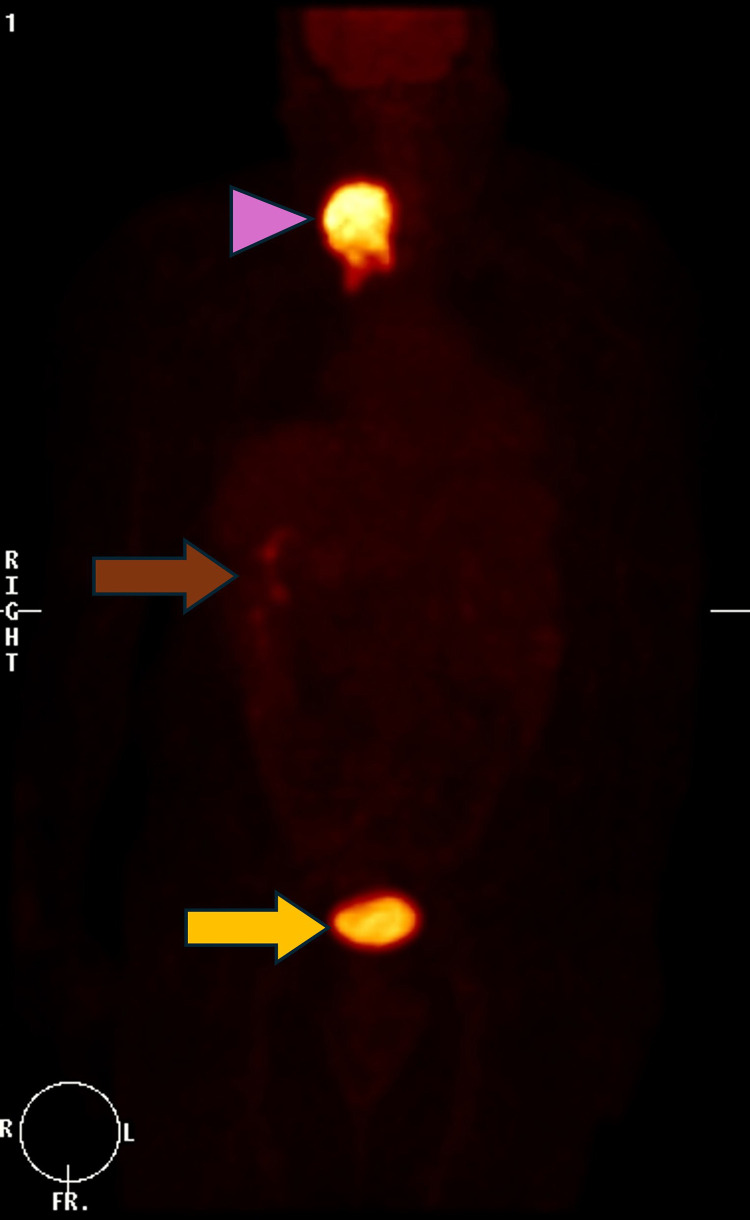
Whole body F-18 FDG PET shows a large area of intense uptake in the right neck (pink arrowhead). There is mild uptake in the region of the gallbladder fossa in this patient with chronic liver disease (brown arrow). Physiologic excretion of radiotracer is seen in the urinary bladder (yellow arrow).
